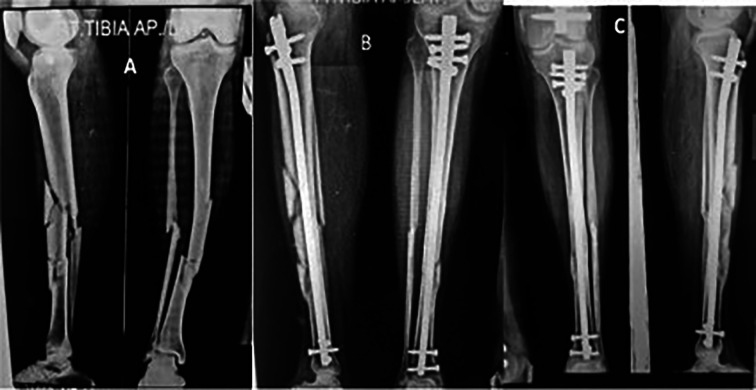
(A) Segmental comminuted fracture tibia, (B) post op x-ray with suprapatellar nail and (C) nine months follow up. Union of fracture.
